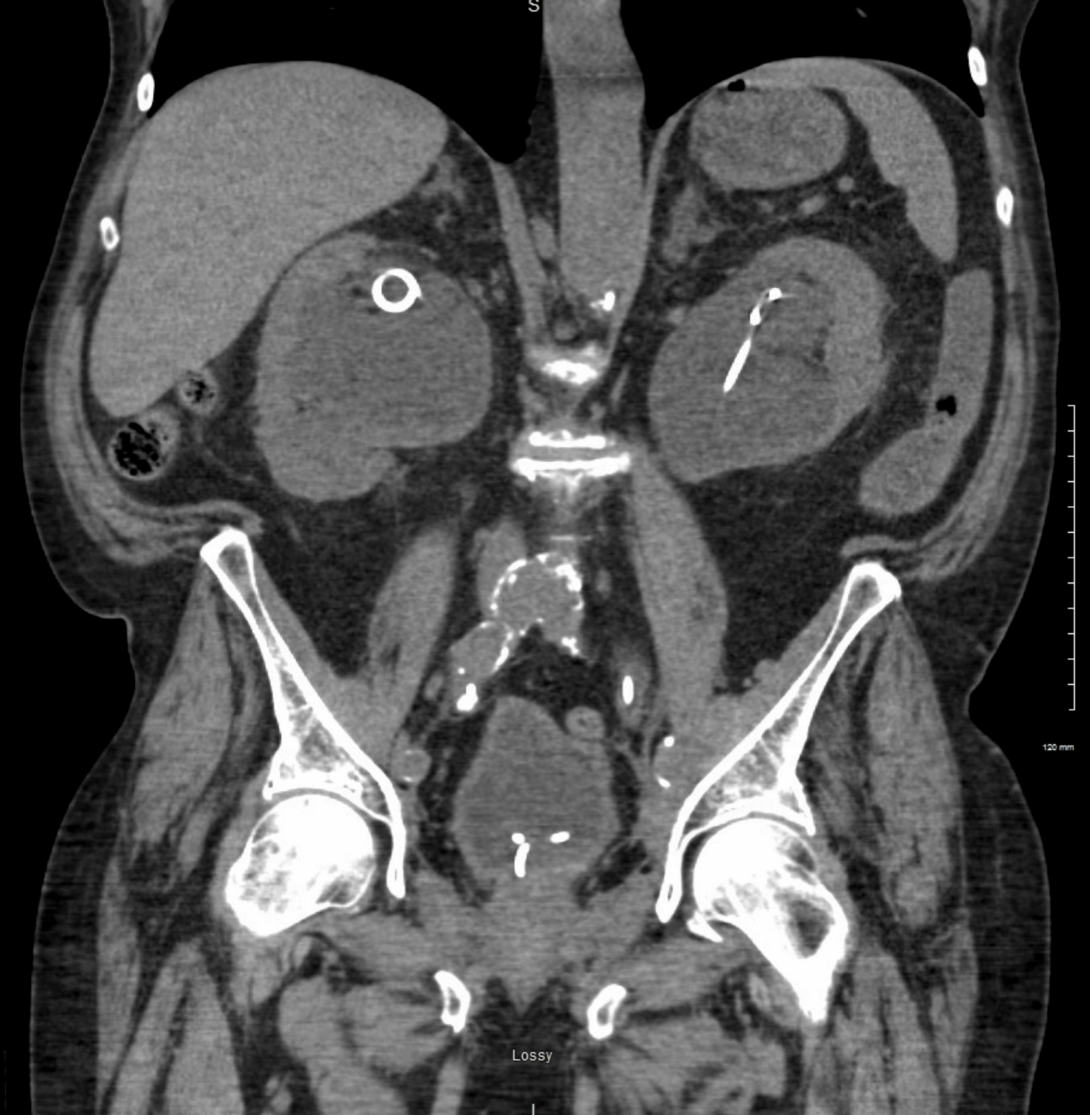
CT scan showing severe bilateral hydroureteronephrosis and the presence of ureter stents
